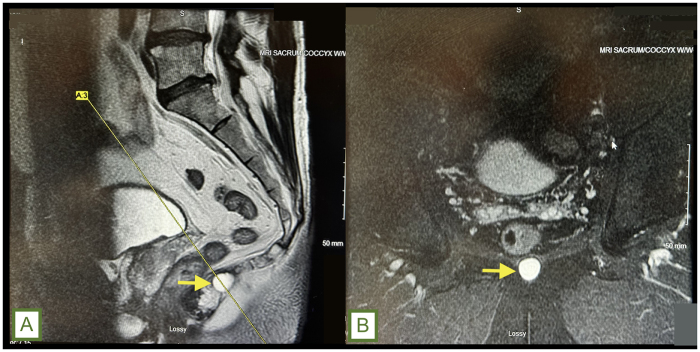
(A) Sagittal section of T2-weighted MRI and (B) coronal section T2 STIR MRI of the sacrum and coccyx showing a cyst in the retrorectal space (yellow arrow).
